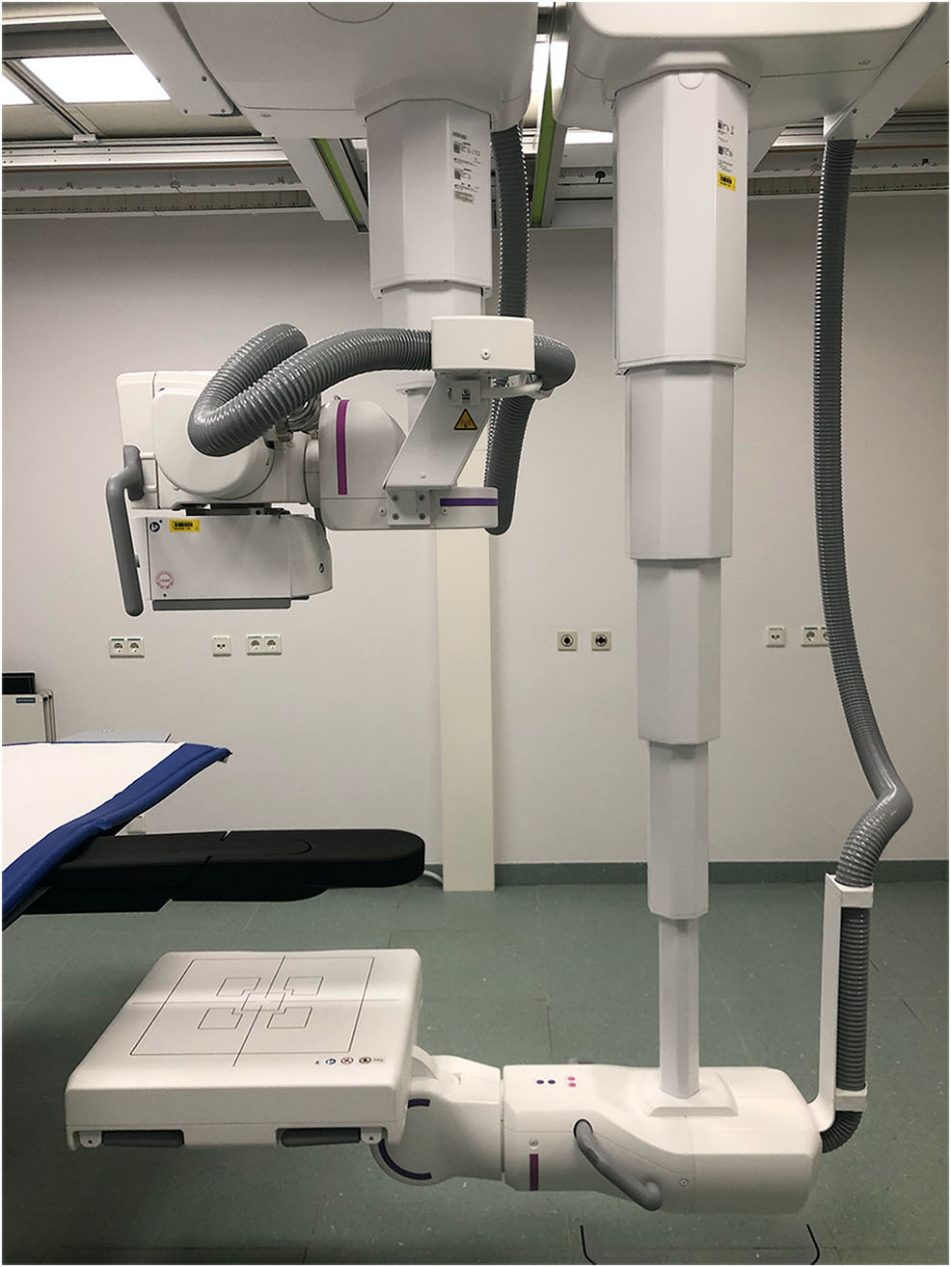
Display of the tableside scan position for wrist imaging with the twin robotic x-ray system
